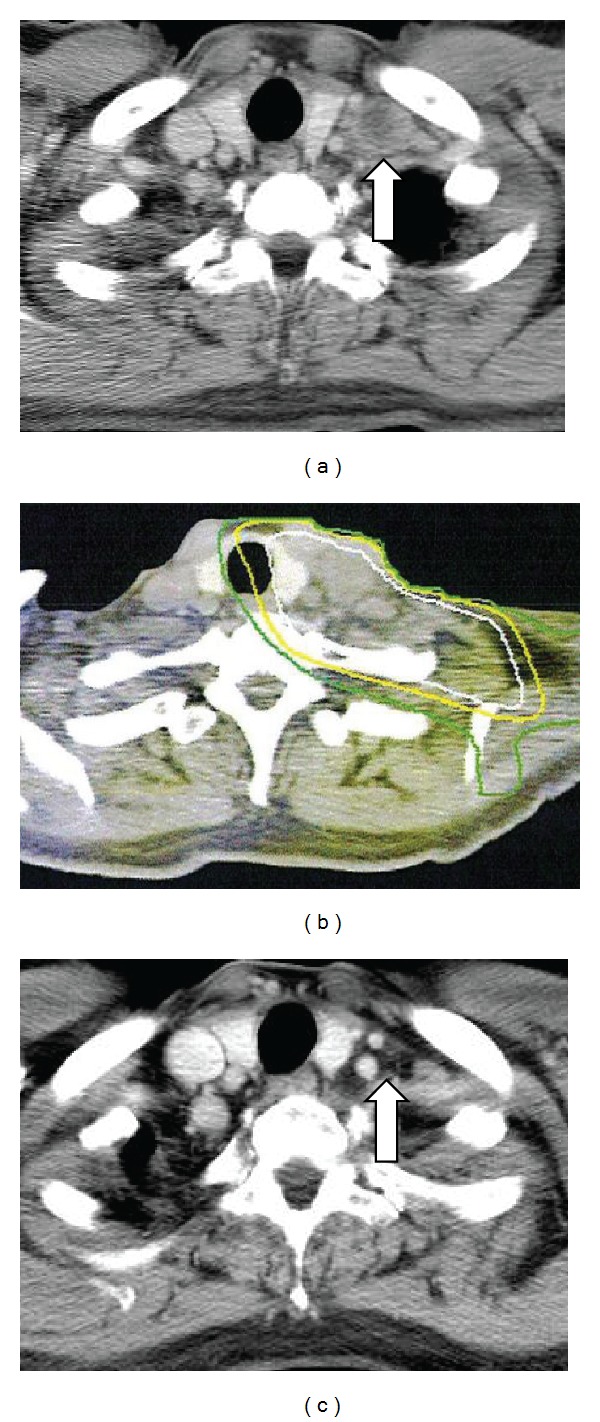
(a) CT of left supraclavicular lymph node metastases (arrow) before SBRT. (b) Dose distribution made with 4 coplanar beams for conventional radiotherapy. Isodose lines shows total doses (in 29 fracions) of 50 Gy, 40 Gy, and 30 Gy, respectively, from innermost area. (c) CT at 1 months after the RT, showing complete response of the lesion (arrow).
